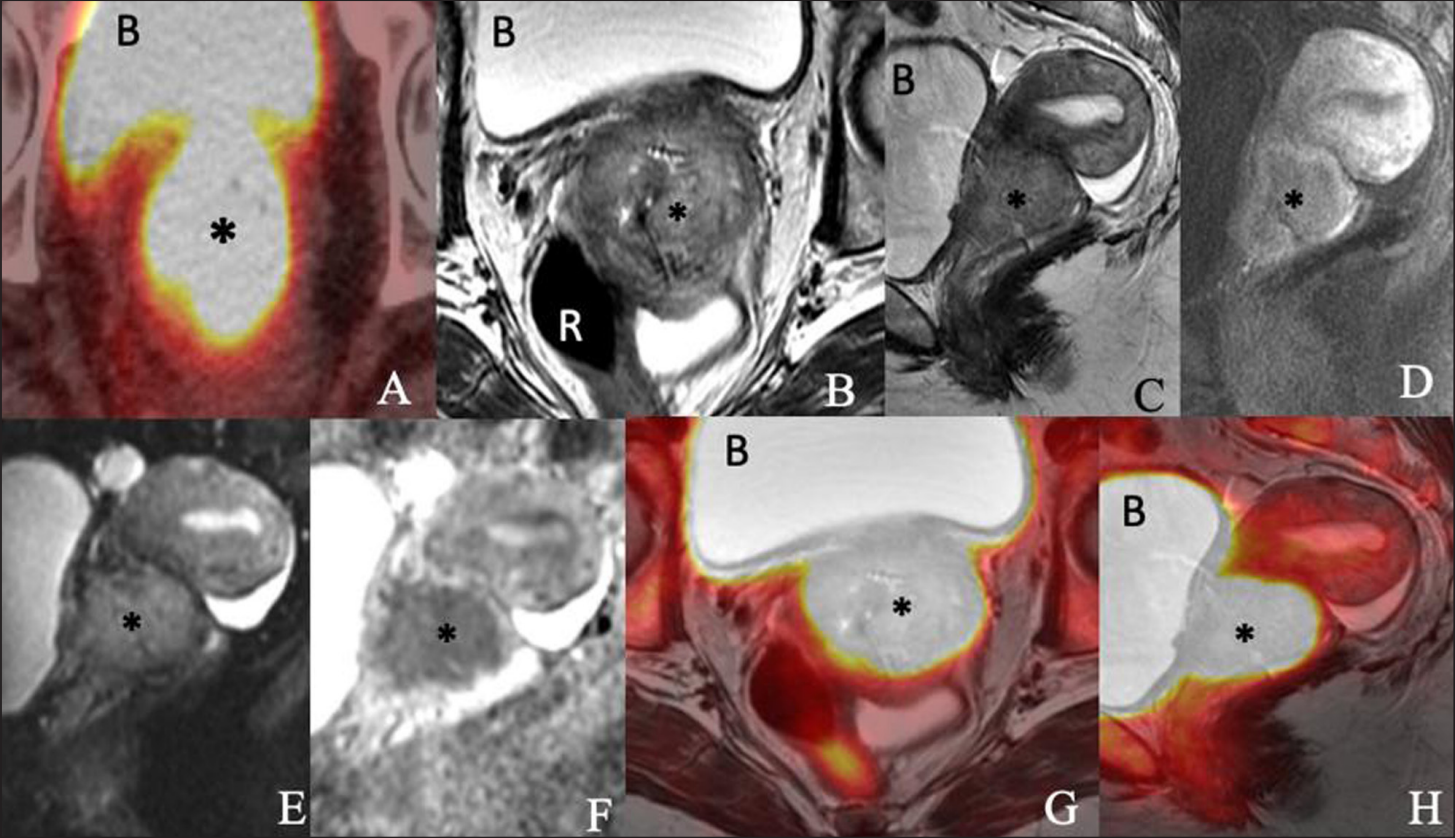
Images of a 76-year-old woman with newly diagnosed squamous cell carcinoma of the cervix consisting of an (A) axial PET/CT, (B) axial T2-weighted, (C) sagittal T2-weighted, (D) sagittal postcontrast T1-weighted, (E) sagittal diffusion-weighted (F) sagittal apparent diffusion coefficient map of MRI, (G) axial T2-weighted PET/MRI and (H) sagittal T2-weighted PET/MRI images show a 5.4 × 4.6 × 4.1-cm enhancing FDG-avid cervical mass (*) invading the parametrium and extending into the vaginal fornices and lower uterine segment. The mass exhibits restricted diffusion. The bladder (B) and rectum (R) appear to be uninvolved.
